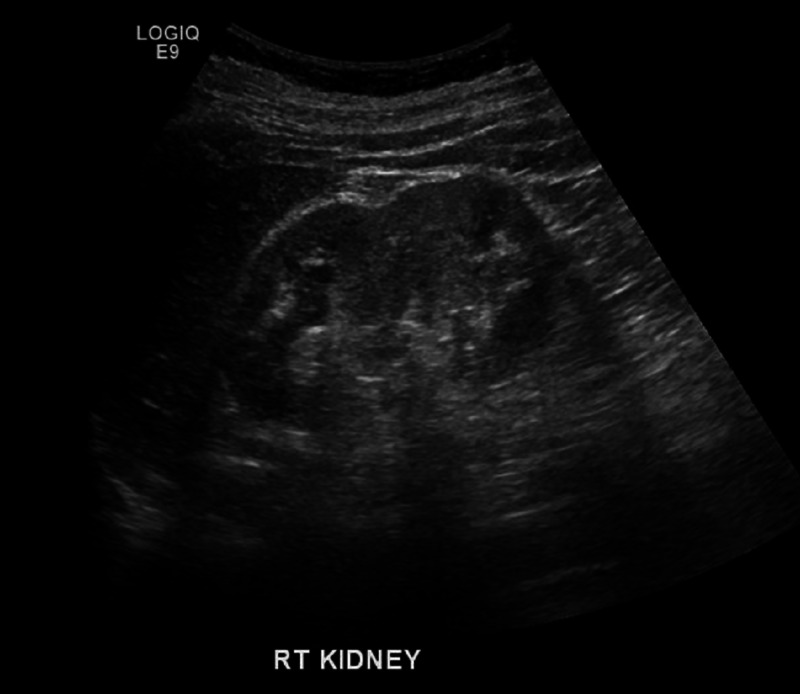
Follow-up ultrasound after three months showing total resolution of perinephric hematoma.
